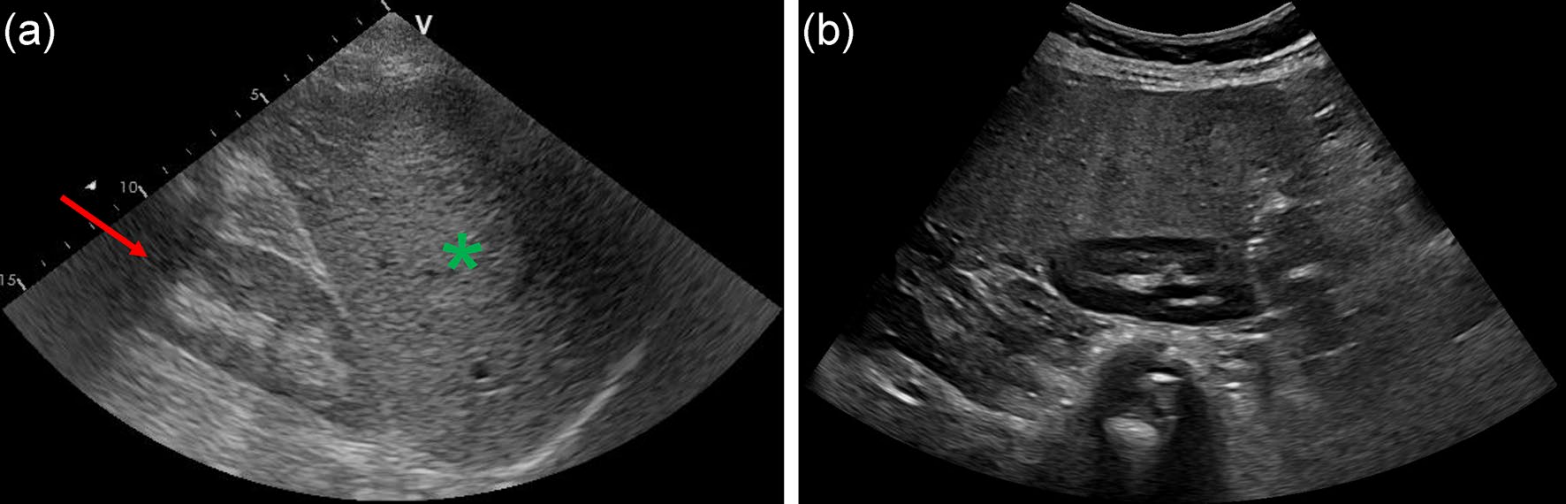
Example of representative B-mode ultrasound images from the public and private datasets. (a) Steatosis grade 0 (3% hepatocytes with steatosis) from the public dataset shows the liver (asterisk) and right kidney (arrow). (b) 73-year-old female with non-alcoholic steatohepatitis with steatosis grade 3 from the private dataset shows moderate activity at the stage of cirrhosis.
